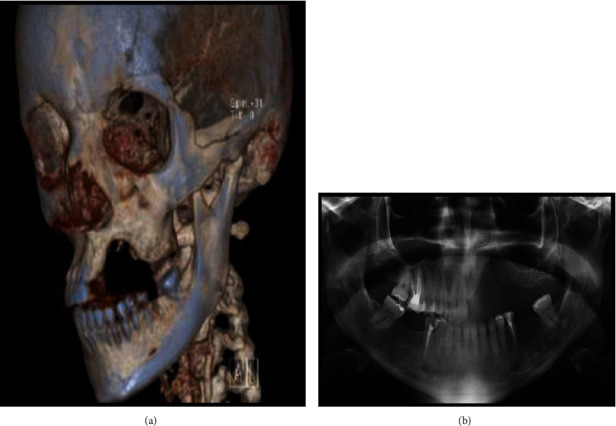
(a) Three-dimensional computed tomography showing partial bony defect of the left maxillary bone. (b) Preoperative panoramic radiography showing a large radiolucent area at the left side of the maxilla.
